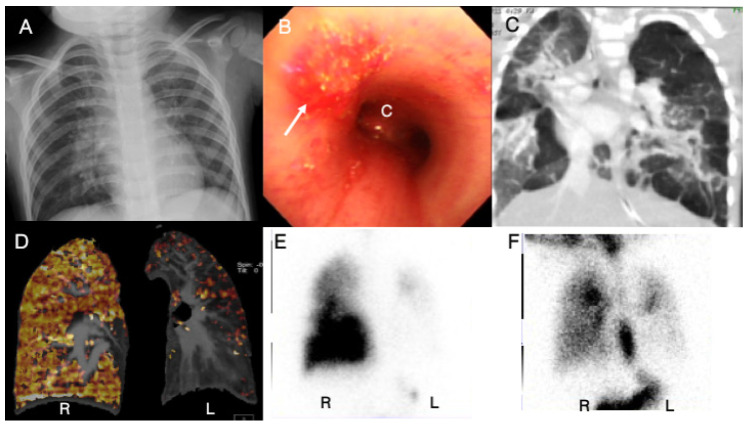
A 3-year-old boy presenting with hemoptysis and dyspnea was diagnosed with Swyer–James–Macleod syndrome and pulmonary hypertension. Chest radiography revealed a unilateral hyperlucent lung (A); a bronchoscopy revealed several erosions and bleeding from the trachea (arrow) (B); coronal-view high-resolution computed tomography of the chest revealed regional ground-glass opacities and focal emphysema, particularly in the left upper lobe (C); dual-energy computer tomography revealed areas of decreased lung attenuation, associated with decreased pulmonary blood vasculature in the left lung (D); perfusion scintigraphy revealed marked defects in left lung fields (E); and ventilation scintigraphy revealed a marked defect corresponding to the previous lab tests (F). C, carina; R, right side; L, left side.
